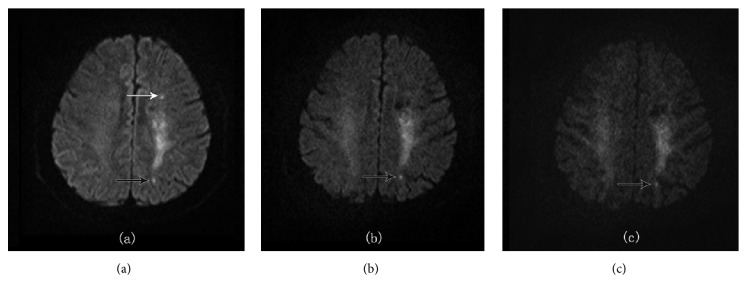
A 47-year-old male patient with acute cerebral infarctions. (a), (b), and (c) were the ss-DWI image with b-values 1000, 2000, and 3000. Two lesions were found at b1000 image. The parietal lesion (black arrow) kept the signal while the b-value was increasing, which should be an infarction lesion. However the frontal lesion (white arrow) decreased the signal while the b-value was increasing, which should be a demyelinating lesion. Noise looked more pronounced in b3000 than b2000.
